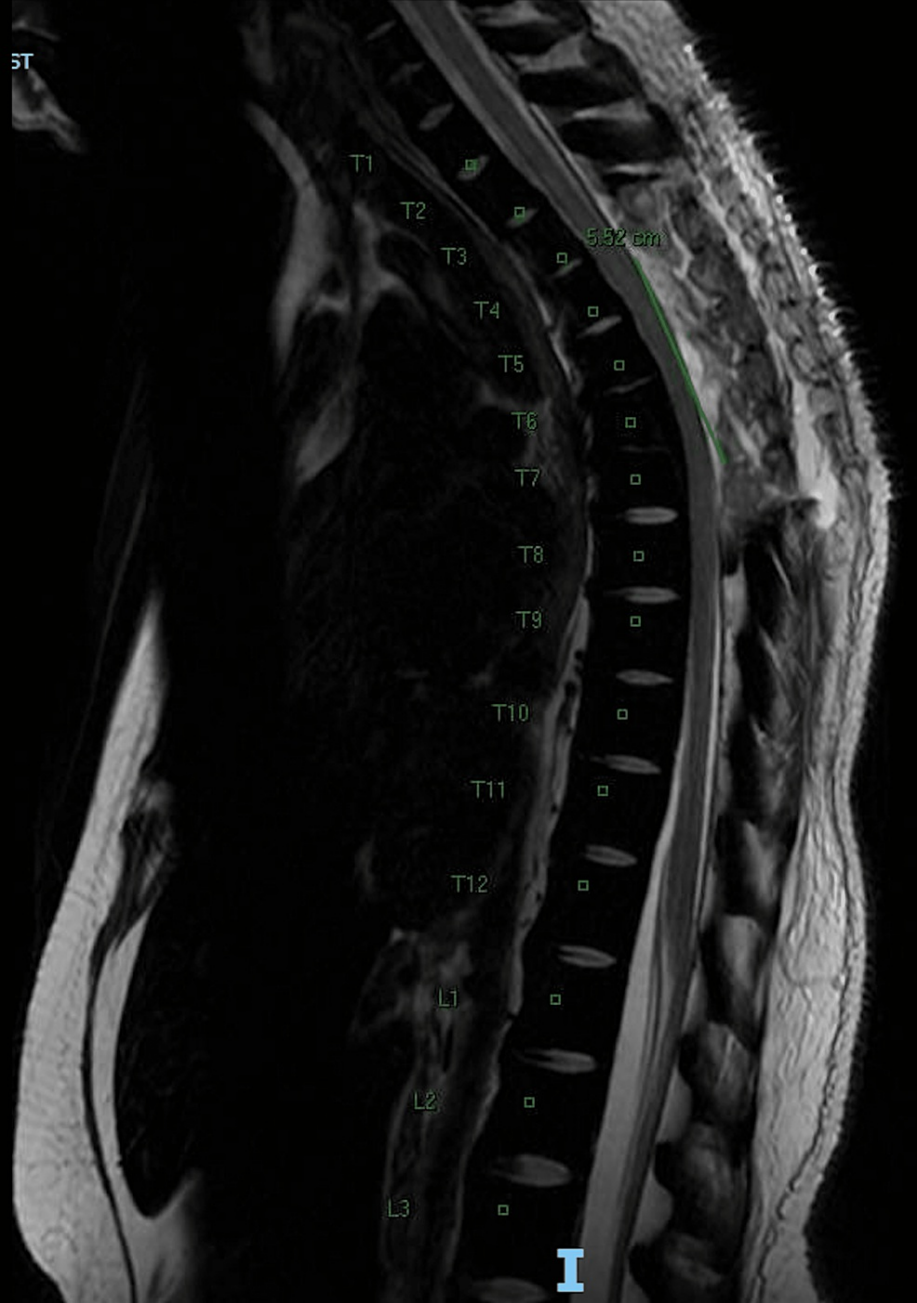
Thoracolumbar MRI post laminectomy.
